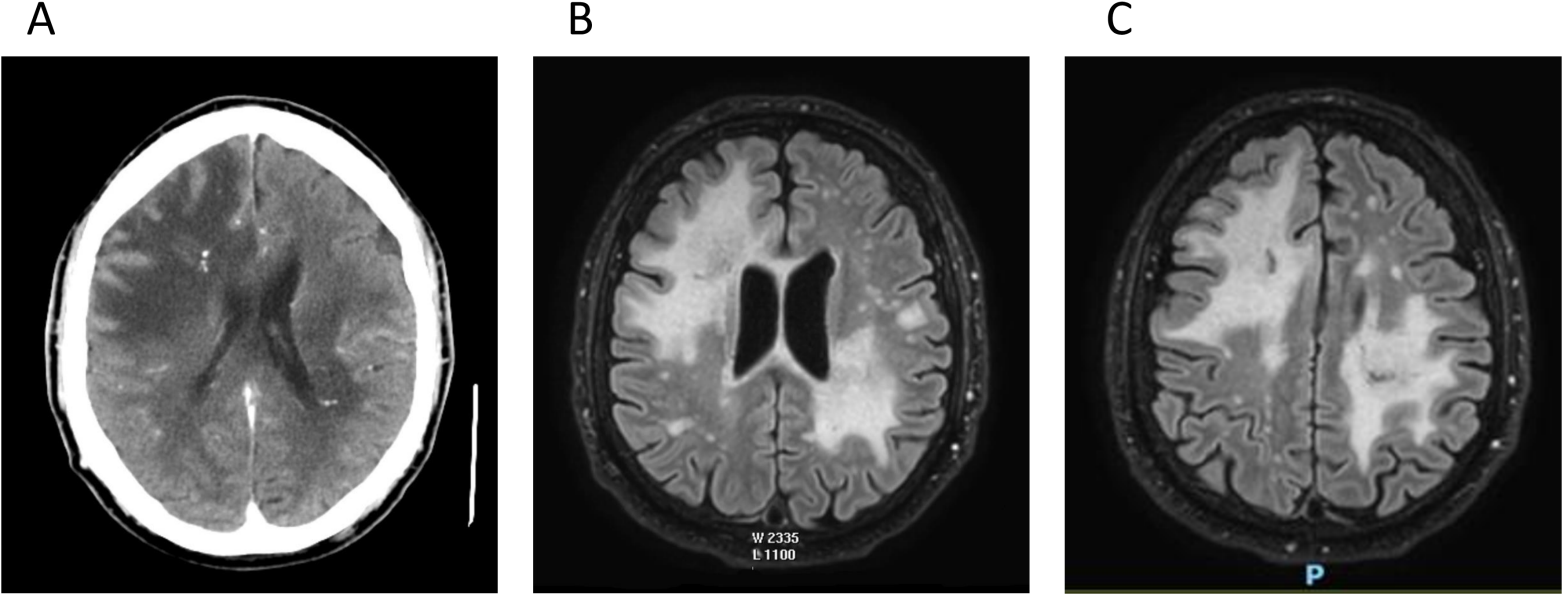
Computed tomography (A) and magnetic resonance imaging (B, C) reveal lesions affecting both the superficial and deep white matter in both cerebral hemispheres. The lesions exhibited faint ring enhancement and focal calcifications were notably present.
